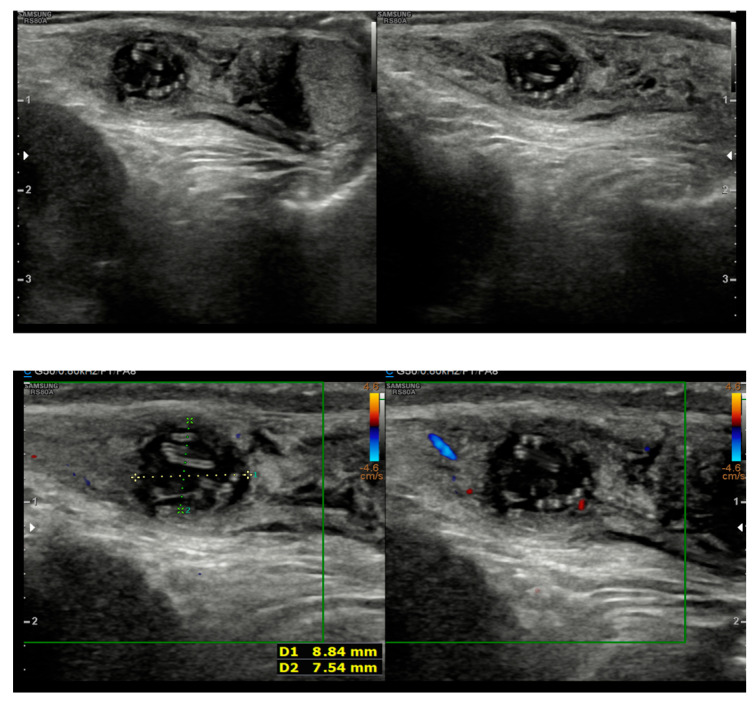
Follow-up ultrasound demonstrating a hypoechoic nodule containing linear hyperechogenic lines.
